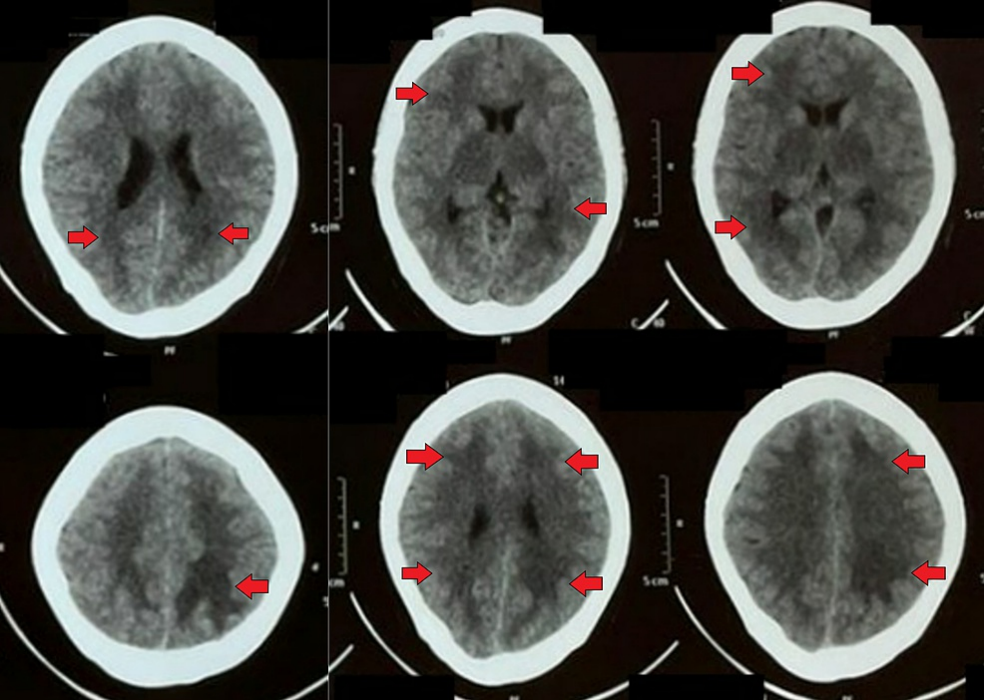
Non-contrast computed tomography of the brain demonstrating diffuse cerebral edema (red arrows)
